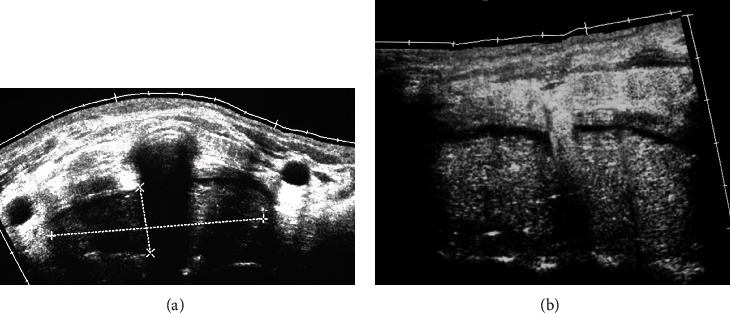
(a, b) Transversal and longitudinal images of ultrasound depicting a serosanguineous fluid collection at the cervical spine in a patient that underwent cervical spine surgery.
